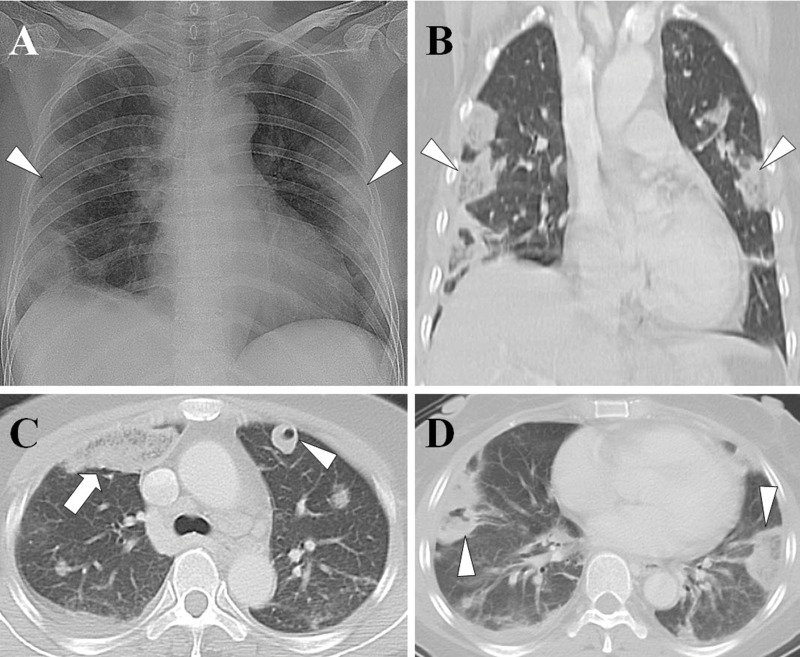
Tricuspid valve infective endocarditis with septic pulmonary emboli. (A) A chest radiograph shows multiple peripheral patchy opacities in the bilateral lungs (arrowheads). (B) A lung window of a coronal CT scan shows multiple peripheral wedge-shaped opacities (arrowheads). (C) A lung window of a cross-sectional CT scan shows a ground-glass opacity in the right upper lobe (arrow) and a nodule with cavity in the left upper lobe (arrowhead). (D) A peripheral wedge-shaped opacity with central necrosis in the right lower lobe (arrowhead) and a peripheral wedge-shaped opacity without central necrosis in the left lower lobe (arrowhead). The patient was a 50-year-old woman whose blood cultures were positive for methicillin-susceptible *Staphylococcus aureus*.
